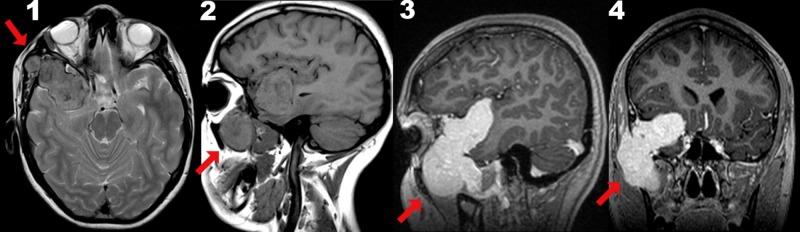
Magnetic resonance imaging Intracranial lesion from the Silvian Valley to the anterior temporal region, extension to the middle floor of the hourglass-shaped skull base, and the right extracranial temporal fossa Views. Left to right: 1 Transversal, 2 Sagital A, 3 Sagital B with gadolinium, 4 Coronal with gadolinium Red arrows 1-4: *An intracranial lesion can be observed from the Silvian Valley to the anterior temporal region, extension to the middle floor of the hourglass-shaped skull base, and right extracranial temporal fossa, with the remodeling of the posterior wall of the maxilla.* 3 and 4: *Intimate contact with lateral wall of the orbit in its intracranial portion, showing enhancement with gadolinium.*
